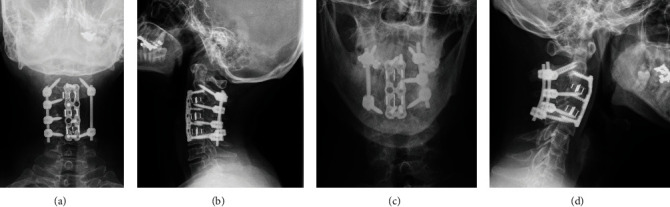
Follow-up. (a, b) a.p. and lateral radiographs of the cervical spine of case 1 at 12 months postoperatively. (c, d) a.p. and lateral radiographs of the cervical spine of case 2 at 4 months postoperatively.
